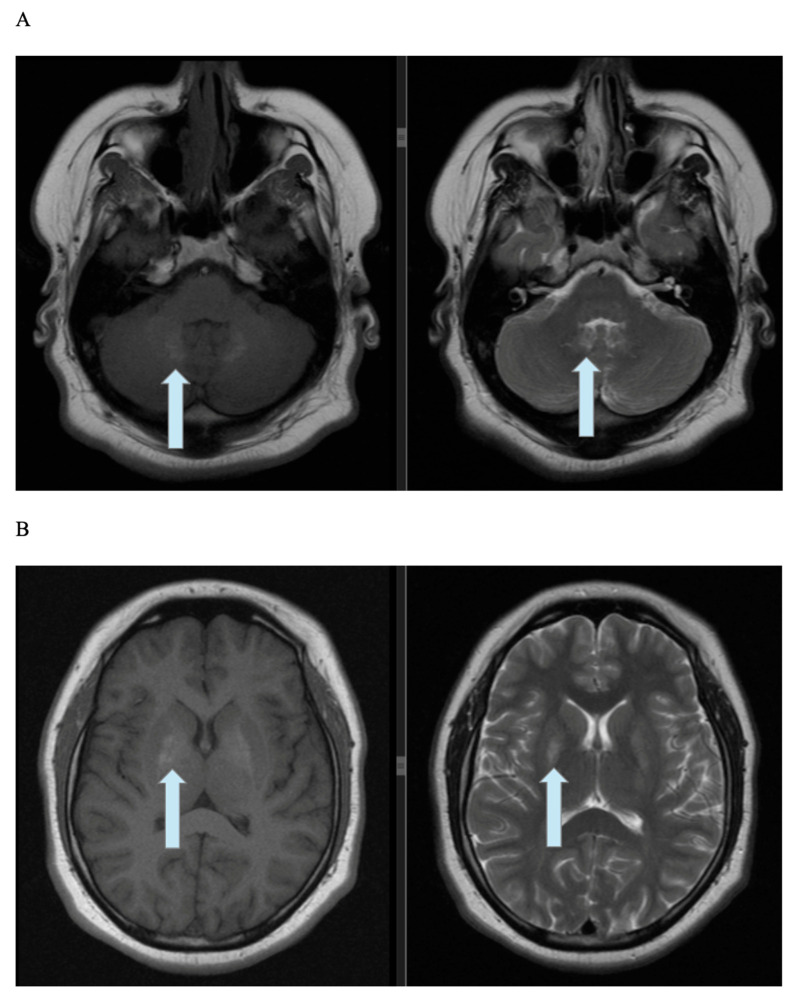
(A) T1WI and T2WI MRI images showing symmetrical hyperintensity in the region of the dentate nuclei. (B) T1WI and T2WI MRI images showing bilateral hyperintensity in the patient’s globus pallidus.
